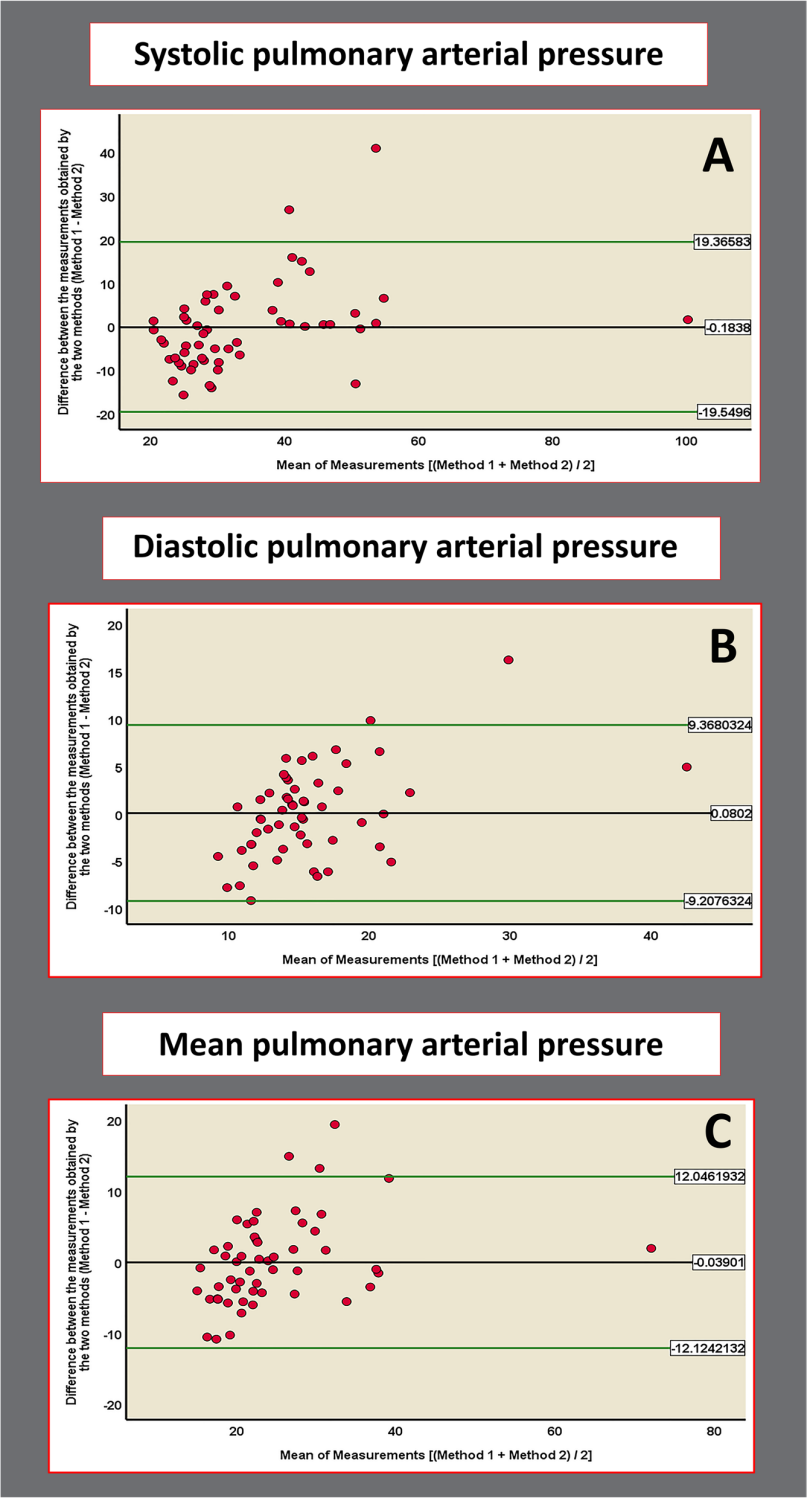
Bland–Altman plots for (A) systolic pulmonary arterial pressure, (B) diastolic pulmonary arterial pressure, and (C) mean pulmonary arterial pressure. The y-axis represents the variation between measured and calculated pressures (method 1, cardiac catheterization; method 2, echocardiography), while the x-axis shows the mean of the readings for each patient. The line y = 0 represents perfect agreement. A Points cluster near zero, with < 5% outside the upper/lower 95% confidence intervals. However, there is a trend of points shifting from below to above the mean, indicating a size-related or proportional bias. B No mean offset, < 5% of points outside the confidence interval, but tighter clustering on the right side. (C) Similar to (A), 5% of points outside the confidence interval, with tighter clustering on the right side. A and B show a distinct trend as we progress from left to right along the plots, corresponding to a shift from lower mean measurement values to higher ones. Specifically, there is an increasing number of data points located above the center line in this direction. This pattern strongly suggests the presence of proportional bias, which could be indicative of the overestimation of method 1 or, more accurately, the underestimation of method 2. Since method 1 involves values obtained through cardiac catheterization, it cannot reasonably be considered to be overestimated
